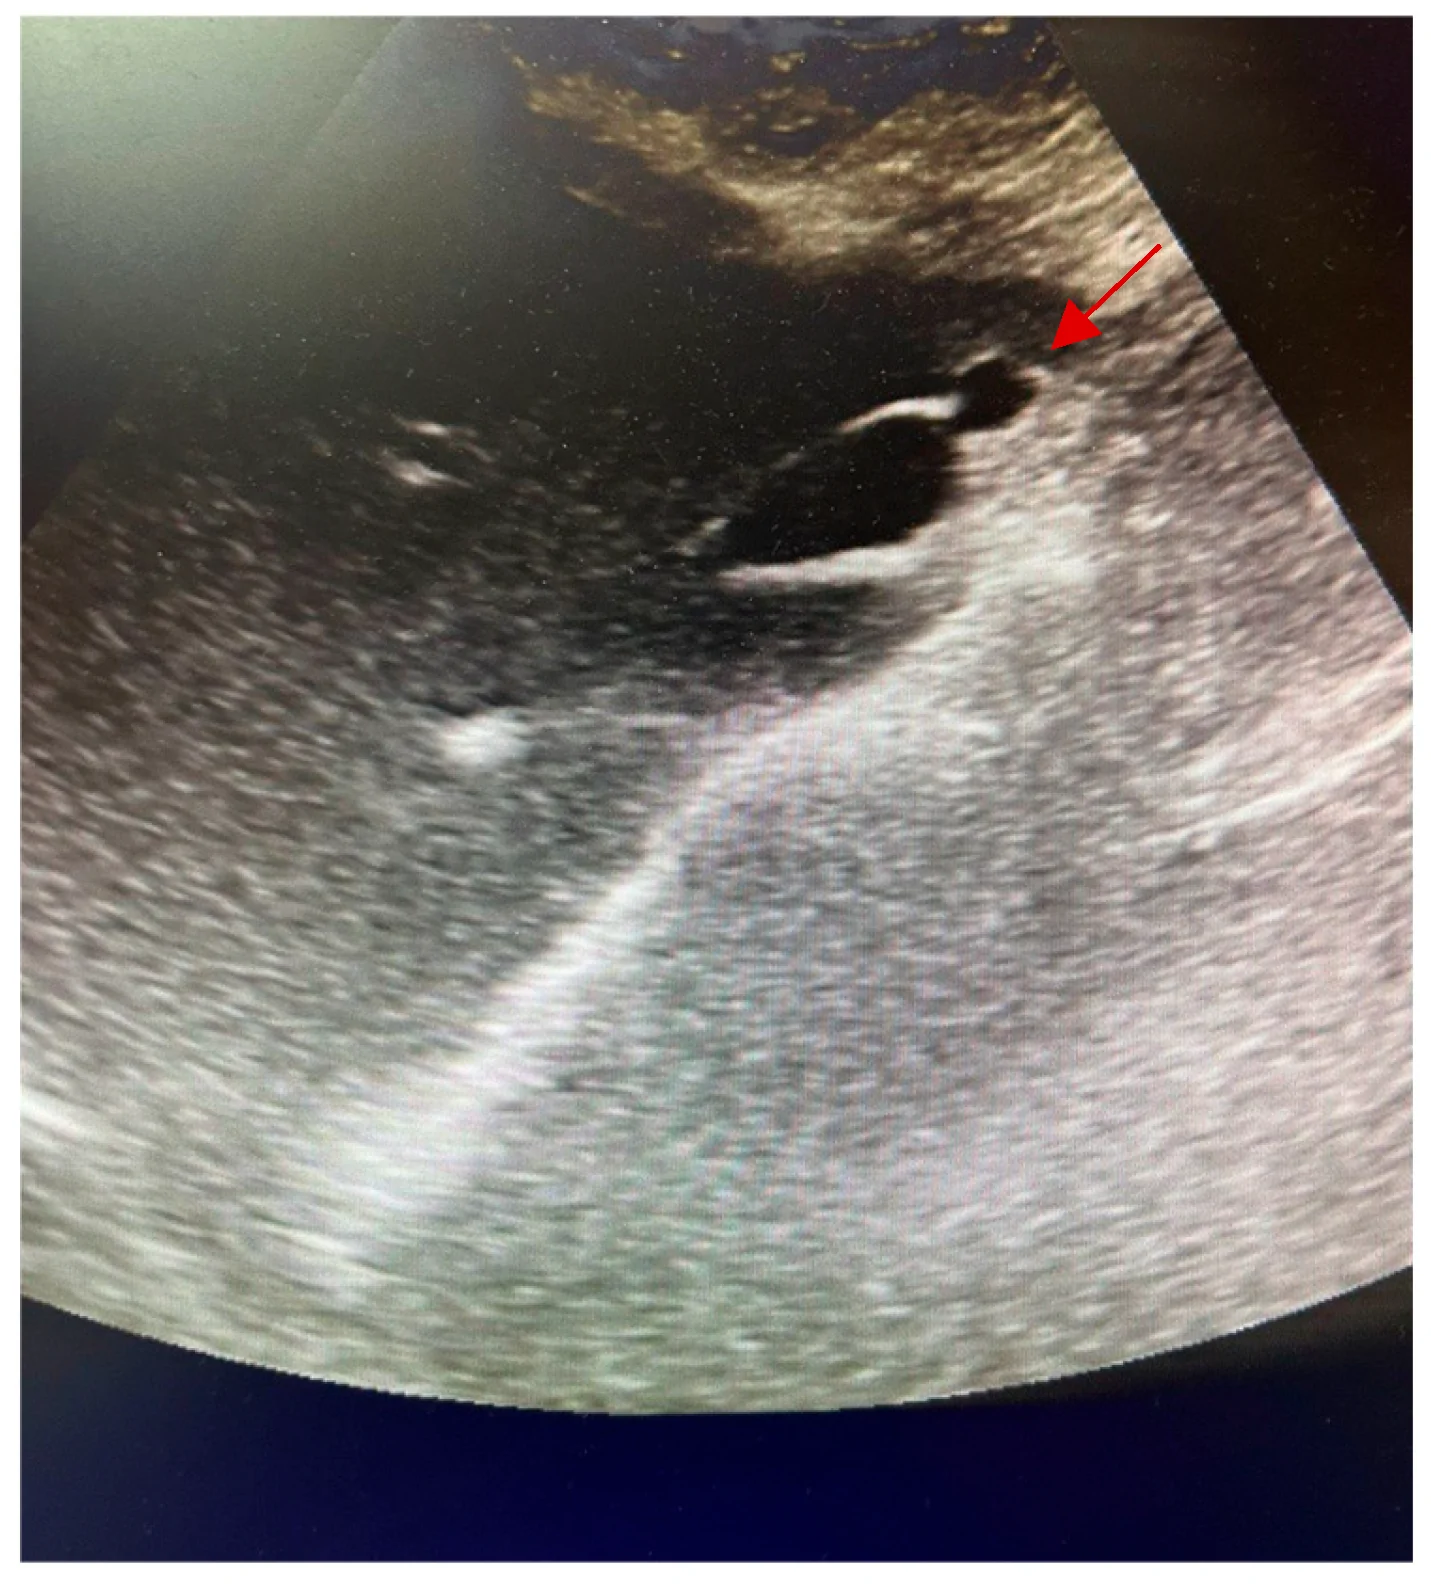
Abdominal ultrasound showing a small cystic outpouching arising from the gallbladder’s fundus (red arrow).
